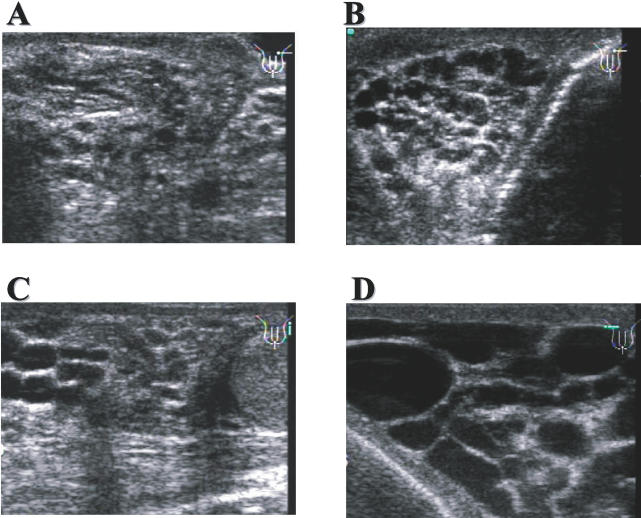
Grading of the Supratesticular Lymphatic Vessel Dilation of Filarial-Infected Patients Displayed by USG Dilation of the supratesticular lymphatic vessels was determined by measuring the largest diameter detectable in the two-dimensional b-mode of a portable ultrasound machine. A grading system was developed to determine the degree of lymphatic dilation as follows: (A) category 1: patients with minimal lymphatic dilation of up to 0.2 cm; (B) category 2: patients with mild dilation from 0.21–0.50 cm; (C) category 3: patients with moderate dilation from 0.51–1.0 cm; and (D) category 4: patients with severe dilation of above 1.0 cm.
